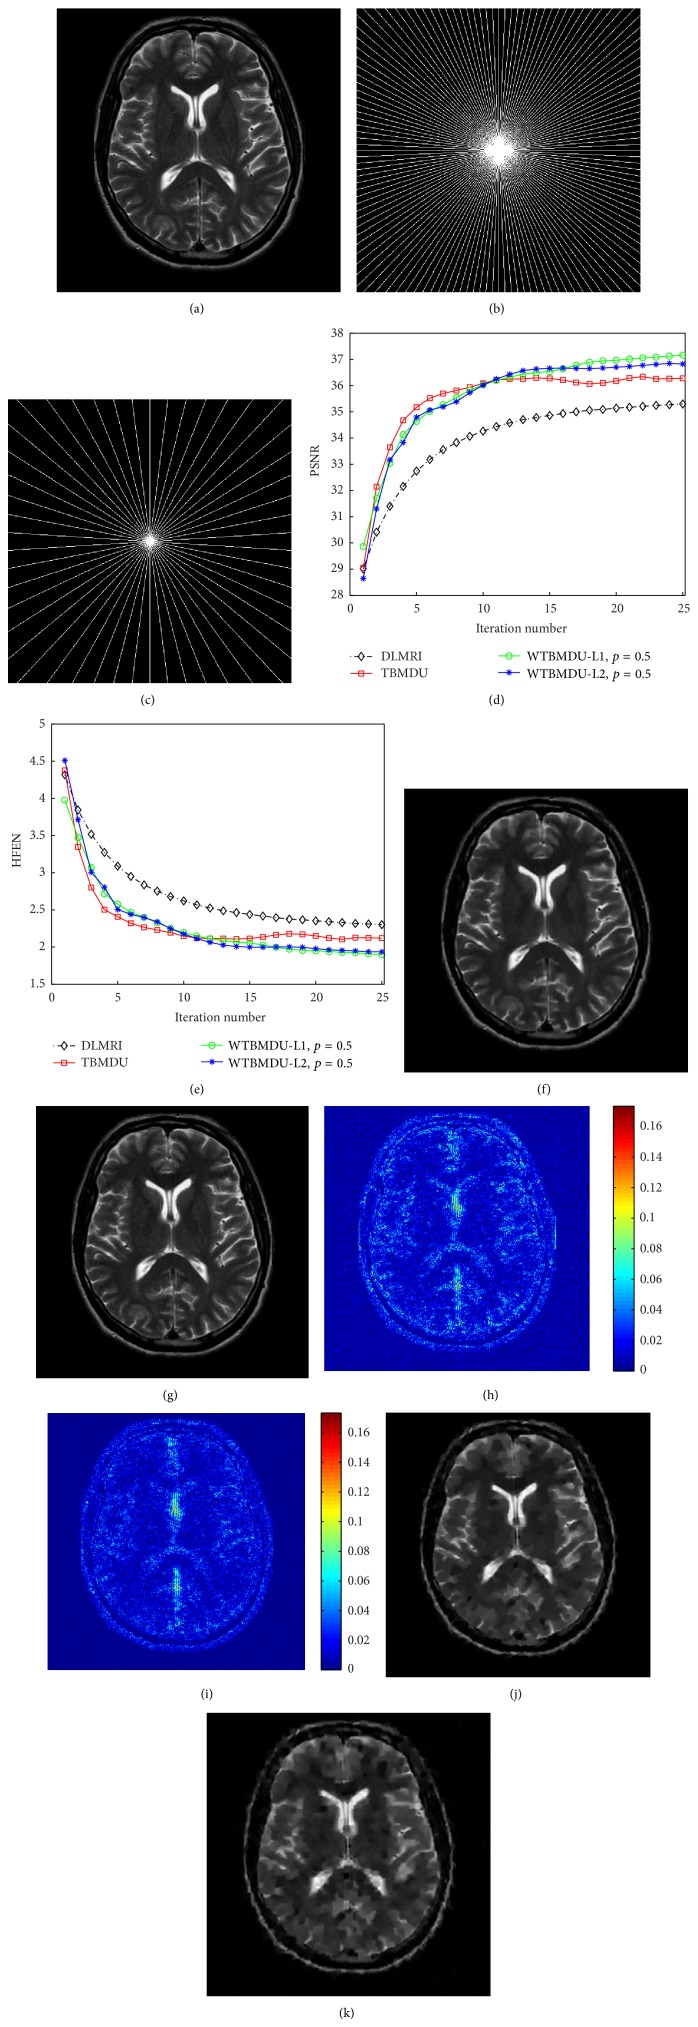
Performance of various algorithms at different undersampling ratios. (a) An axial T2-weighted reference image of the brain. ((b) and (c)) Pseudo radial sampling with 85% and 95% undersampling. (d) and (e) are the PSNR and HFEN versus the number of iterations of DLMRI, TBMDU, and WTBMDU. ((f), (g), (h), and (i)) The reconstruction results and the corresponding error magnitudes of DLMRI and WTBMDU-L1 with *p* = 0.5 at 85% undersampling. ((j) and (h)) The reconstruction results of DLMRI and WTBMDU-L1 with *p* = 0.5 at 95% undersampling.
